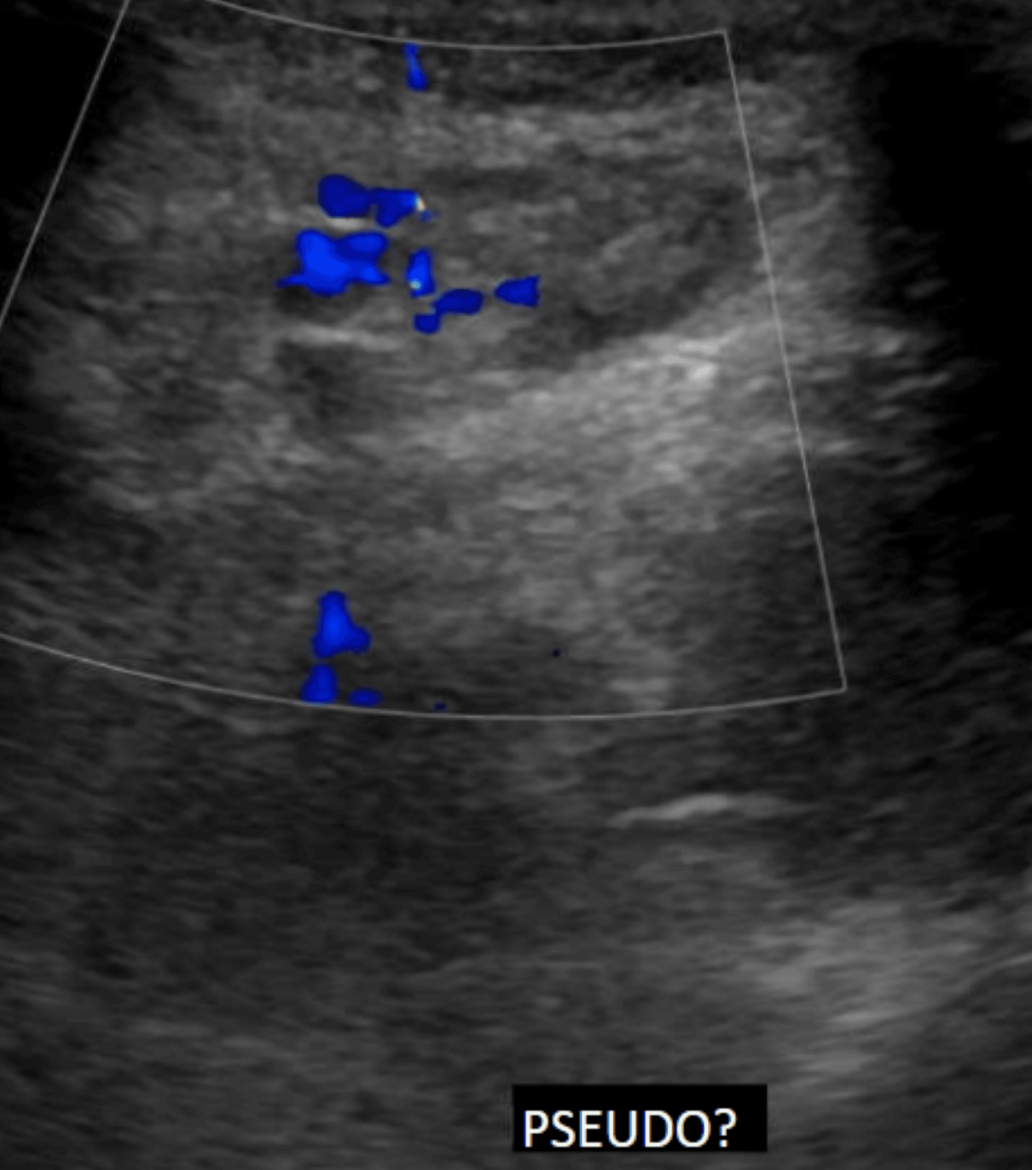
Vascular ultrasound of the right inguinal mass, static image Doppler flow is noted within the mass. Imaging is indeterminate, and a pseudoaneurysm cannot be ruled out.
